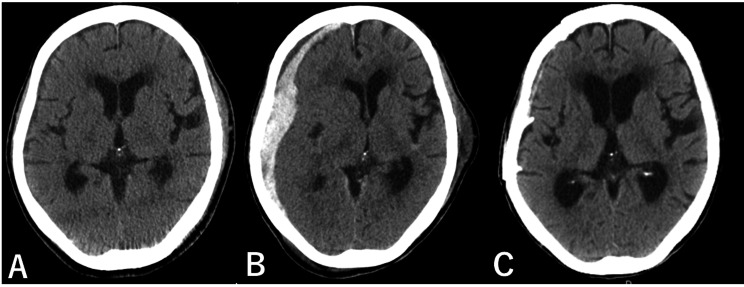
(A) Axial head computed tomography (CT) image on admission. No evidence of intracranial haemorrhage was observed at the time of admission. (A) Axial head computed tomography (CT) image on day 3 post-admission. A right acute subdural haematoma measuring up to 17 mm in thickness was present, associated with a 6.5 mm midline shift indicative of brain herniation. (C) Axial head computed tomography (CT) image on day 67 post-admission. Follow-up axial head CT images on day 67 showed no recurrence of subdural haematoma
